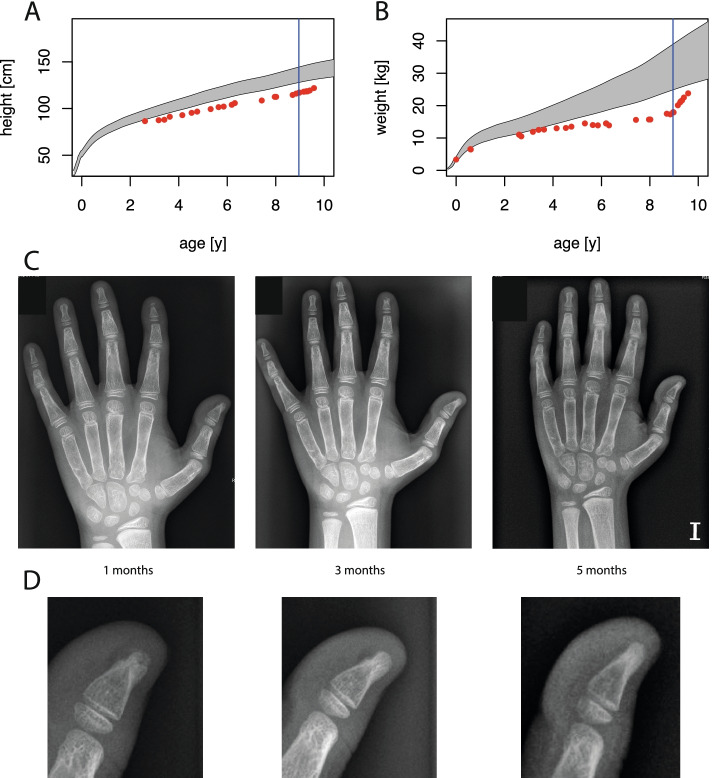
Effects of JAK inhibition on body weight and bone mineralization. A Growth chart showing development of height. The grey area indicates is the 5. to 95. percentiles for height of children in Europe. B Growth chart showing development of weight. The grey area indicates is the 5. to 95. percentiles for height of children in Europe. The dark blue vertical line at 9 years indicates start of treatment. C Series of palmar-dorsal hand X-rays of the left hand showing improved mineralization. The time points after initiation of treatment indicated below images. The scale bar on the lower right corner of panel C is 10 mm and applies to all images of panel C. D Enlarged images of thumb bones showing resolution of acro-osteolysis
